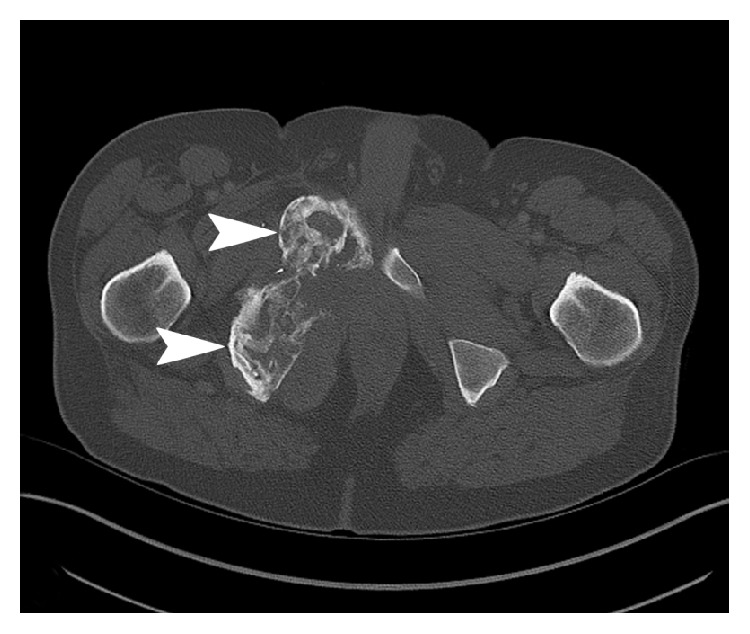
Axial CT of the pelvis (February 2015) showed extensive cortical irregularity and cystic spaces (arrows) of the right inferior pubic ramus (bone window algorithm).
